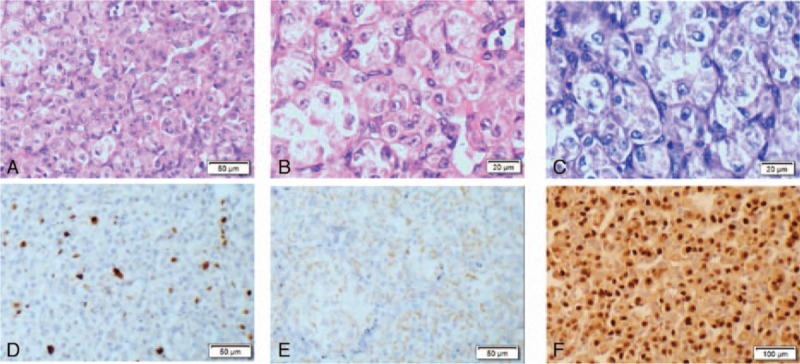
Magnetic resonance imagings (MRI) of the calf. (A) The MRI of the calf before second enlarged resection. (B) The MRI of the calf after radiotherapy. (C) The MRI of the calf at 3-year after radiotherapy.
